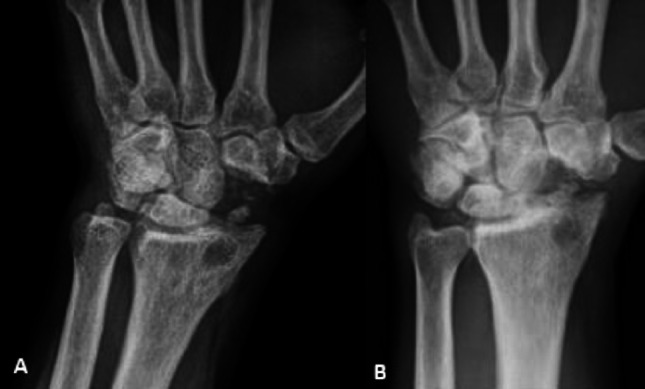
Wrist and hand X-ray in the same patient 8 weeks (A) and 12 years (B) after the procedure
